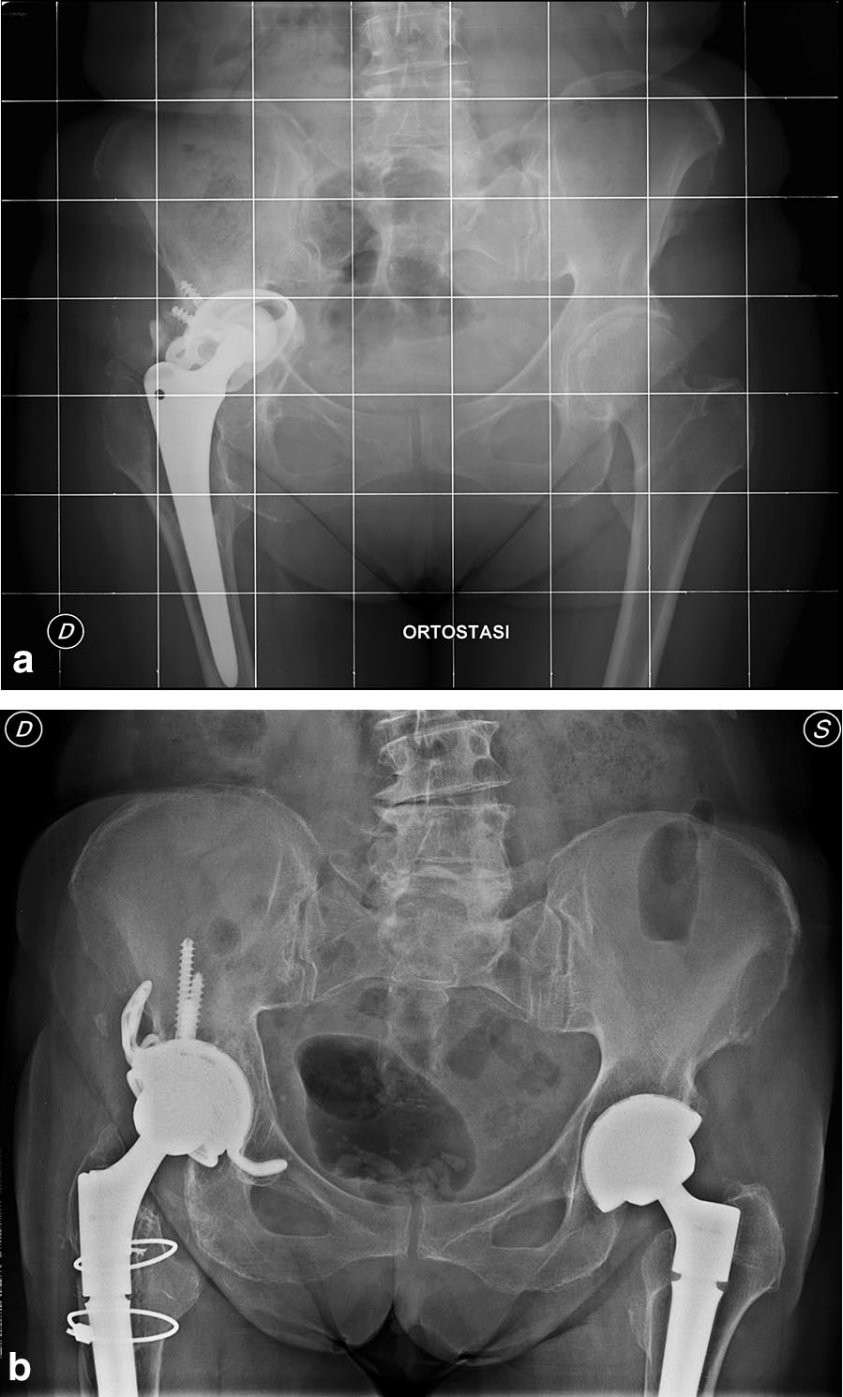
a Preoperative x-rays of cup loosening at right side; b 9 years follow-up after hip revision with Delta TT Revision cup, face changer, and modular stem on right side; 4 years follow-up after THA on left side
